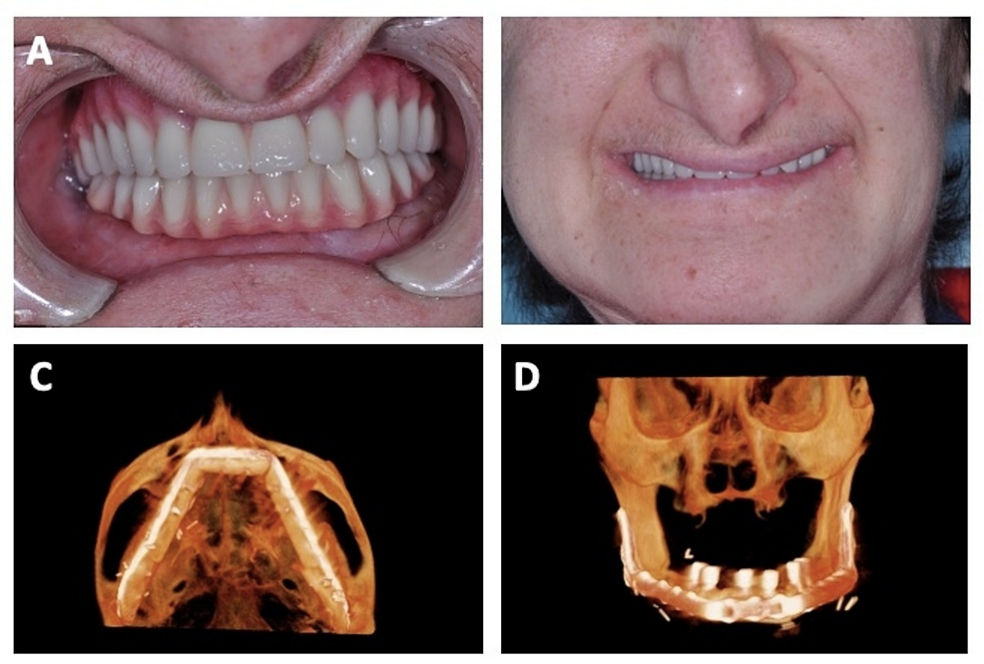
Six months postoperative follow-up with placement of crown prostheses Six-month postoperative photos after placement of crown prostheses into intraoperatively placed dental implants in close-up view showing grossly normal occlusion (A) and with patient smiling. Six-month postoperative CT three-dimensional reconstruction scan from bottom (C) and frontal views (D).
